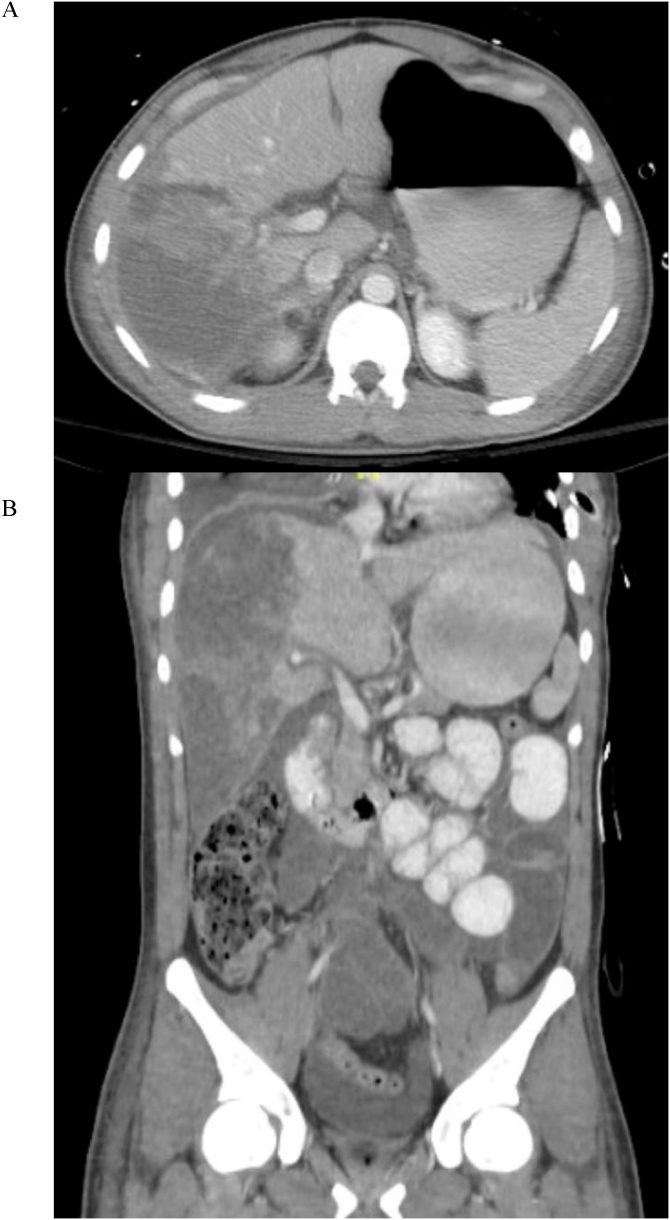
Axial (A) and coronal (B) abdominal CT scans demonstrating AAST grade 5 hepatic laceration with extravasation in segments 5 and 6.
